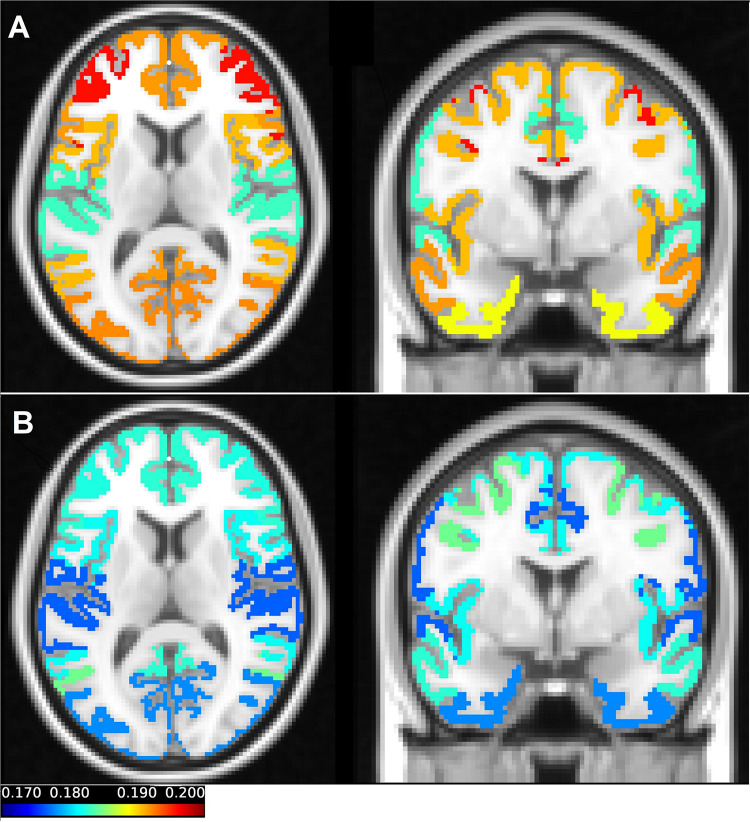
The average entropy values across scans of each of the seven networks in resting state (A) and during a working memory task (B) were mapped on the T1-weighted image. The coronal and axial views were selected in a way that all parcellations can be presented in one figure. In (A) the axial view clearly demonstrates the frontoparietal network, which has the highest value of the entropy during resting state. The somatomotor and limbic networks have low values of the entropy in the resting state. In (B) the dorsal attention network which has the highest entropy during the working memory task can be seen both in the coronal and axial view. Also, in (B) the visual and limbic networks are presented, which have the lowest values of entropy in task.
